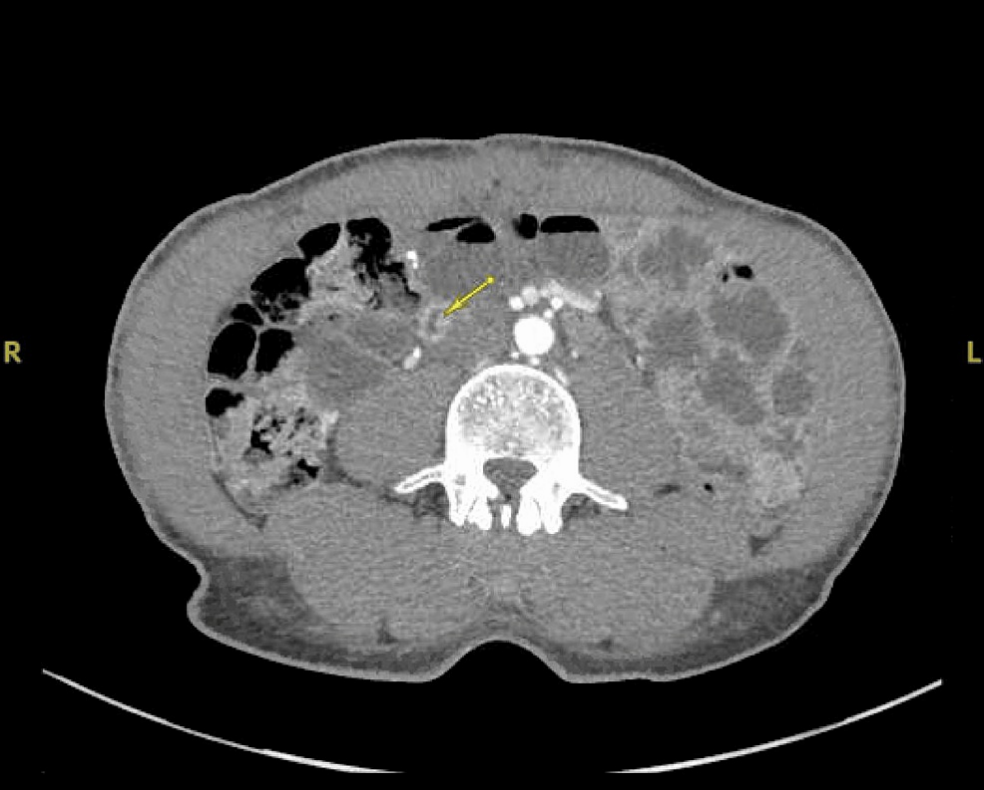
CT small bowel enterography (cross-sectional view) showing soft tissue tract extending from the distal duodenum to the cecum
